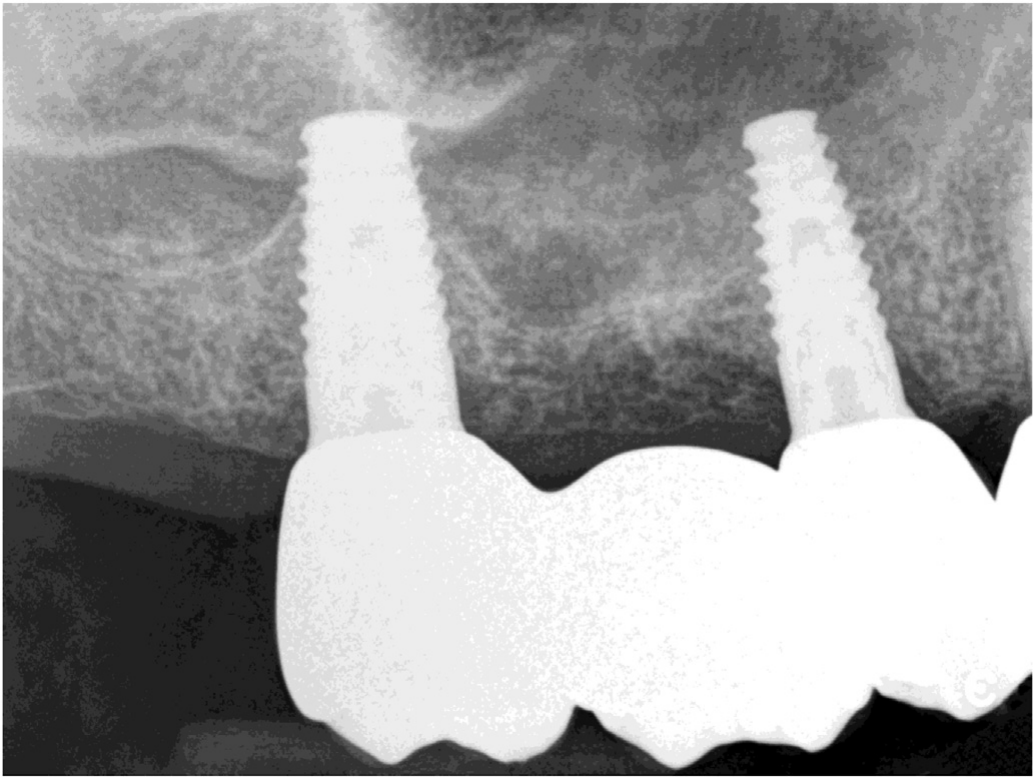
Periapical check X-ray taken with the paralleling technique one year after surgery with the final fixed prosthesis. Ongoing ossification of the elevated bone around the implant apex is evident. The implant in the bicuspid position was inserted with the traditional LMSF technique. The image shows a new elevated line of cortical bone forming between the two implant tips.
